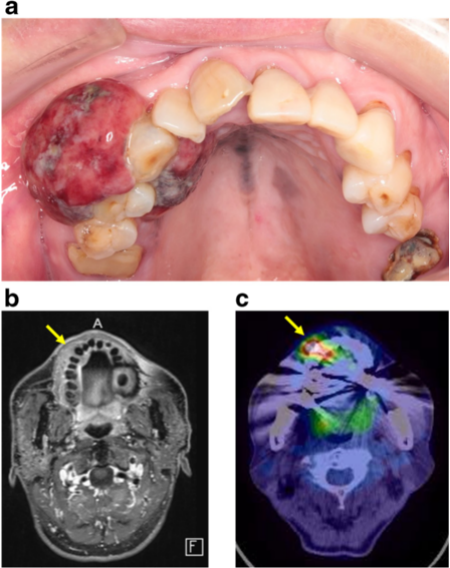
A 66-year-old man with mucosal melanoma of the right maxillary gingiva and palate. (a) Intraoral photograph before carbon ion radiotherapy (C-ion RT). (b) Gadolinium-enhanced T1-weighted magnetic resonance image before C-ion RT. Yellow arrow revealed a mass on the maxillary gingiva (16 × 10 mm). (c) F-18 fluorodeoxyglucose positron emission tomography image before C-ion RT. Yellow arrow revealed abnormal accumulation in the tumor
